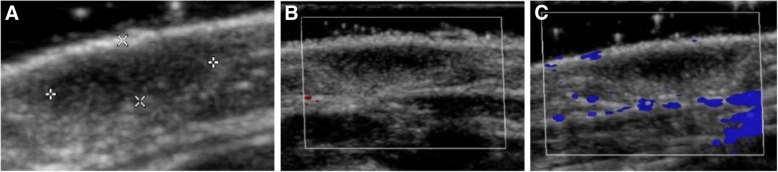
a Ultrasound of cutis/subcutis showed an ovaloid, hypoechoic change in the cutis, diameter 0.8, b and c Colour Doppler image shows no vascularity
